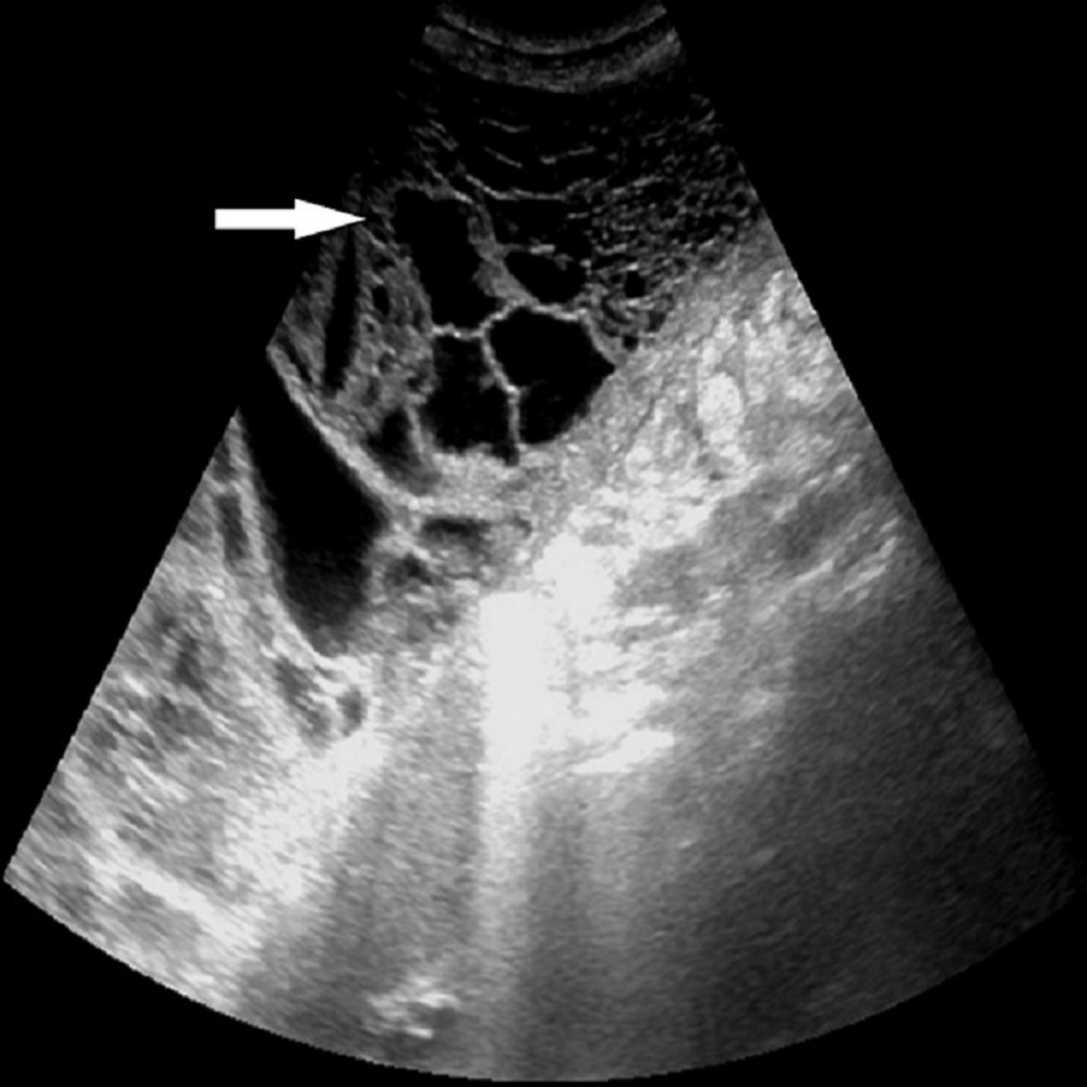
Ultrasound of the abdomen showing multiple fluid-filled loculations
